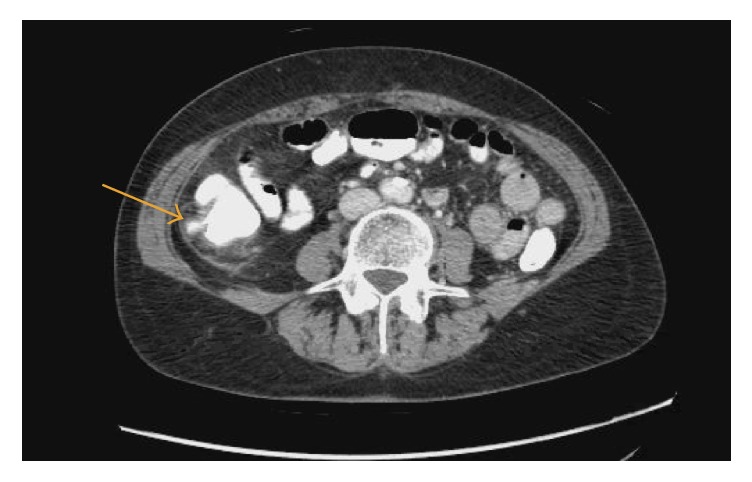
CT scan (axial view) showing an extension of contrast from the right posterior colon, cranial to the appendix.
